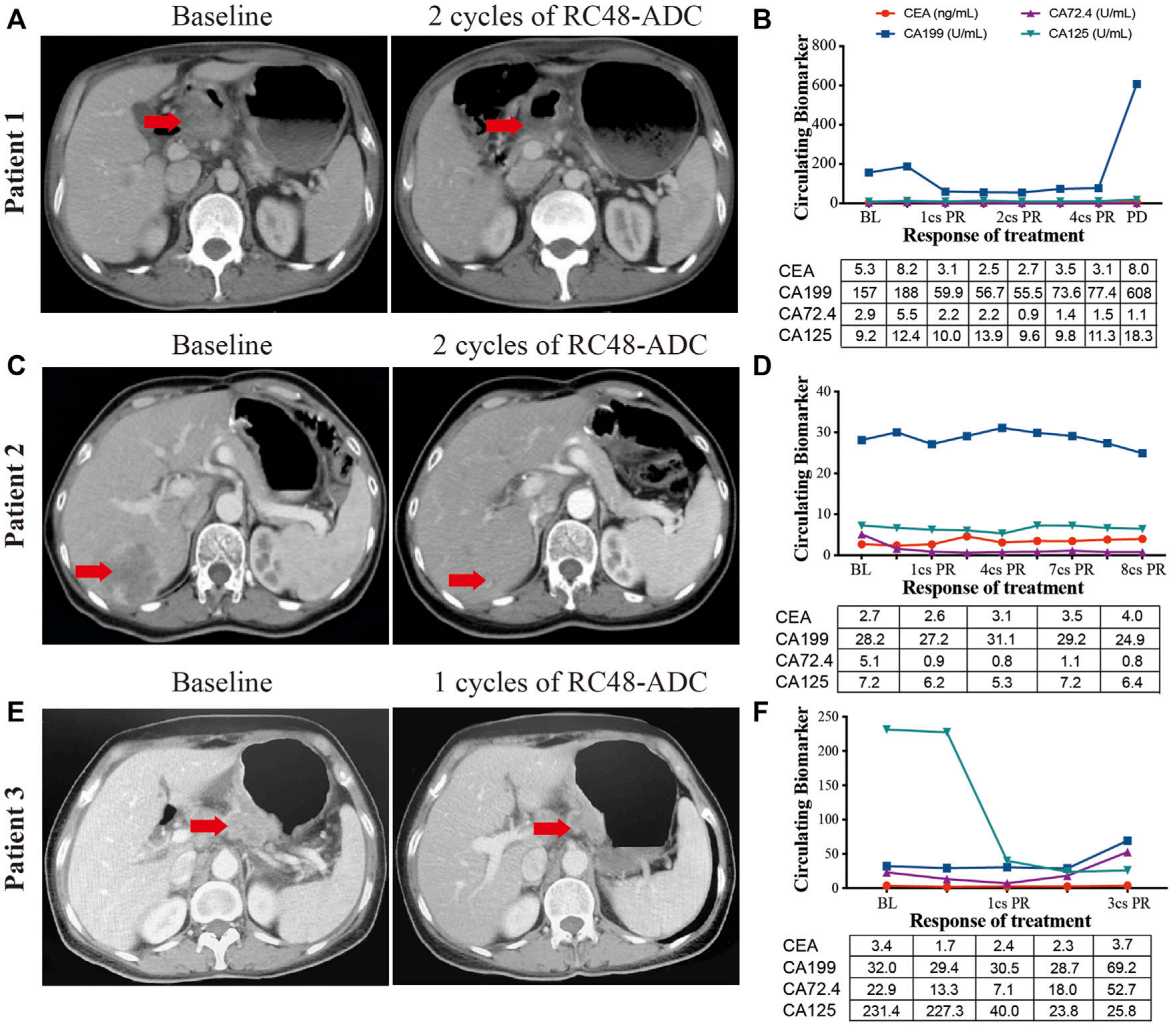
The clinical responses of three human epidermal growth factor receptor 2 (HER2)-expressed advanced gastric cancer (AGC) patients treated with RC48-ADC. (A, C, E) Abdominal CT scans of tumor lesions before and after one or two cycles of RC48-ADC treatment in three patients. (B, D, F) The dynamic change of CEA, CA199, CA72.4, and CA125 during RC48-ADC treatment in three patients. BL, baseline; PR, partial response; SD, stable disease; PD, progressive disease.
